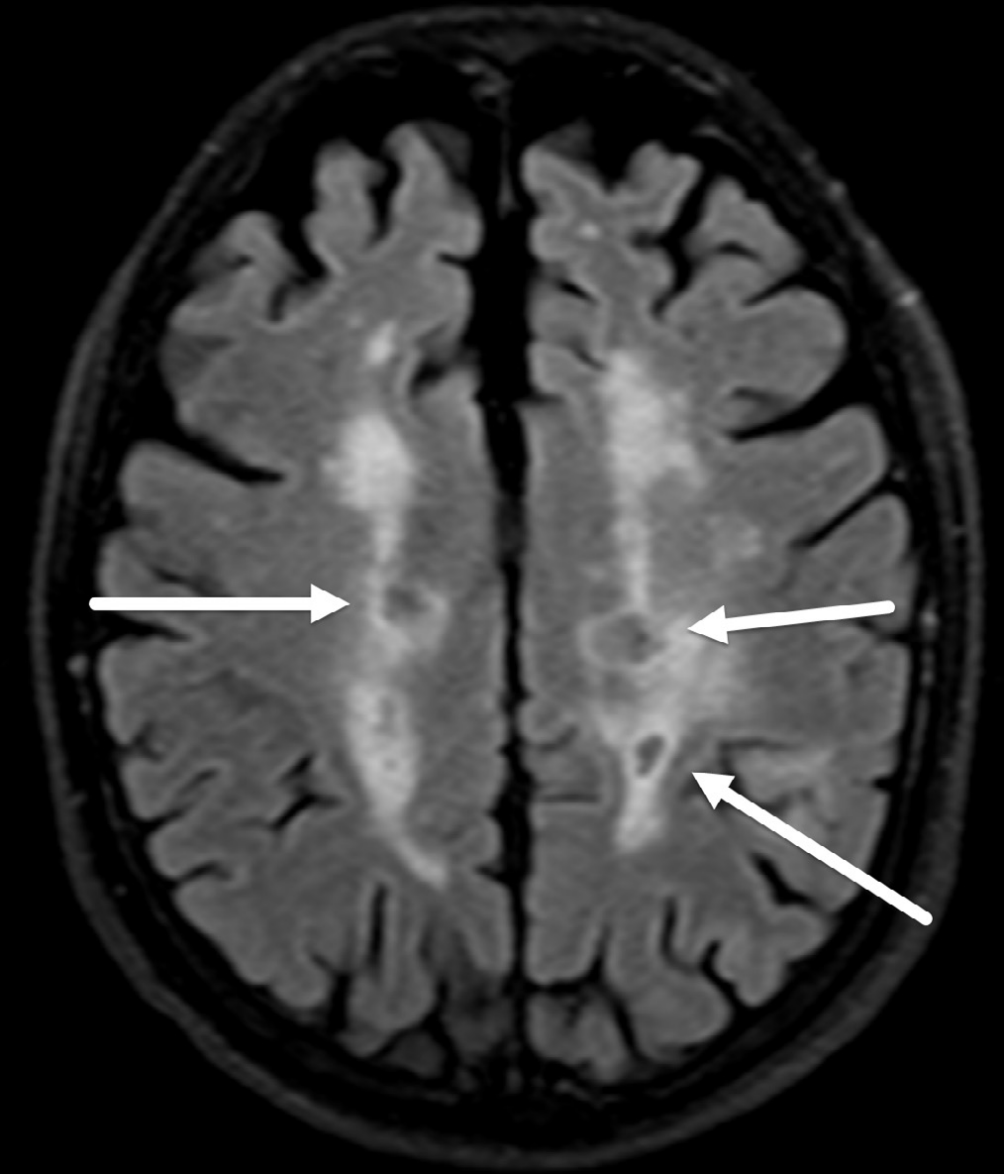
FLAIR signal suppression in T1 black holes in high burden multiple sclerosis. Axial FLAIR MR image shows areas of FLAIR signal suppression in the periventricular lesions (arrows) in a patient with known multiple sclerosis, corresponding to T1 black holes, a finding often seen in patients with severe disease burden. FLAIR, fluid attenuated inversion recovery.
